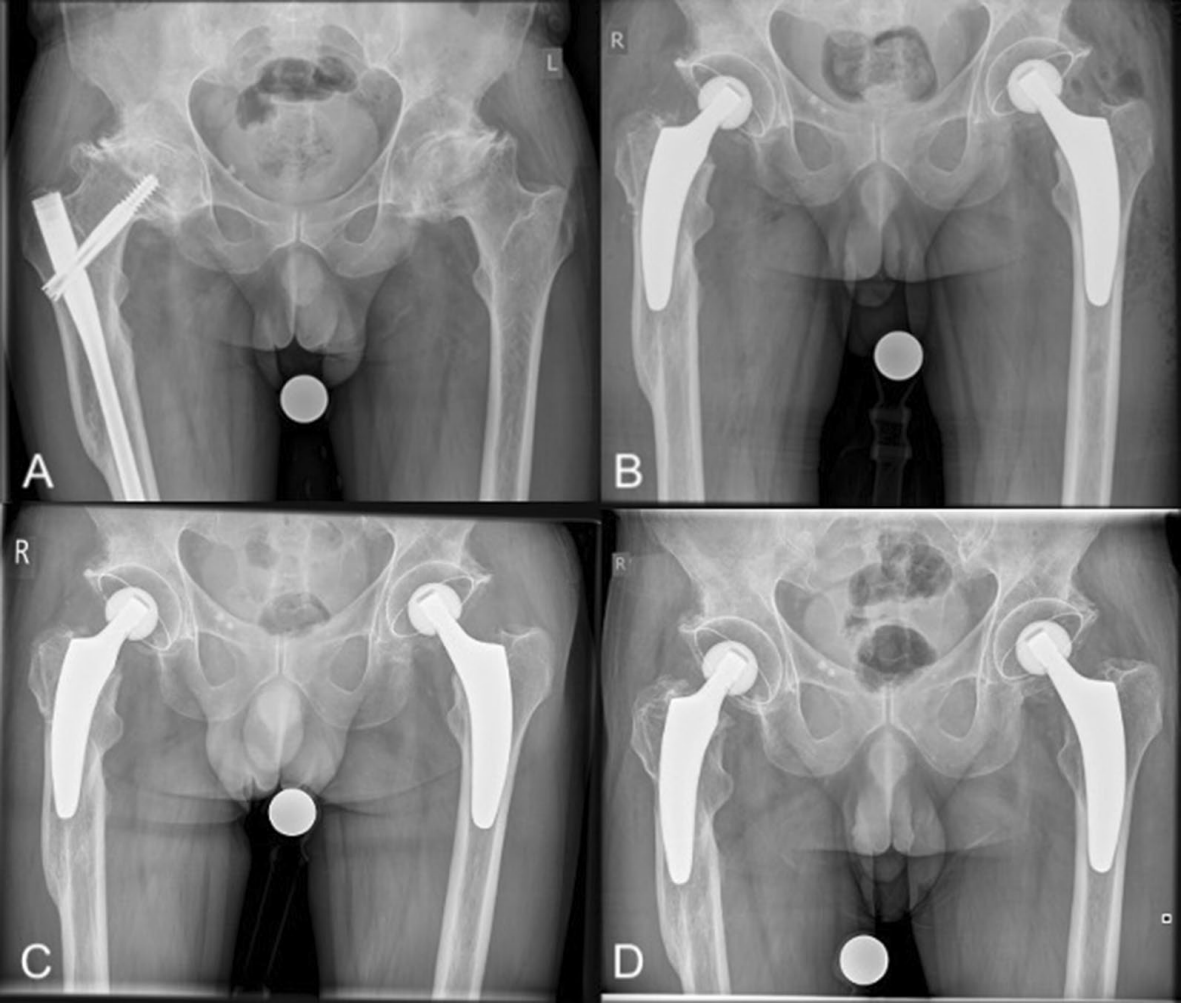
Radiographs of a 57-year-old male patient with secondary osteoarthritis (*right*) and primary osteoarthritis (*left*): A preoperative; B postoperative following one-stage bilateral THA; C 6-week follow-up; D 24-month follow-up (no signs of loosening)
